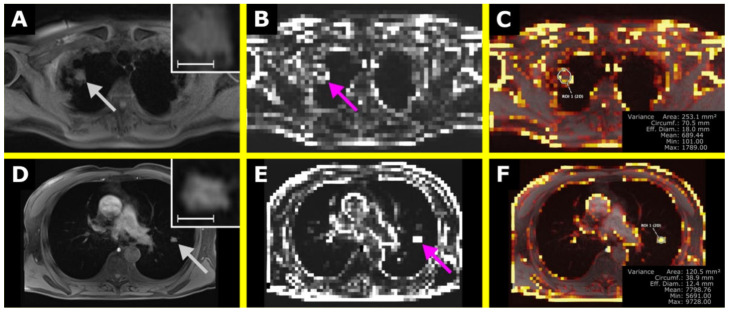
The upper row shows a pulmonary lymphoma manifestation in the right upper lobe with the T1-weighted (T1w) image in (A) and the corresponding map of Variance in (B). In (C), an overlay was created, also showing a ROI to retrieve Variance within the lesion. The lower row shows a non-lymphoma infiltrate in suspected fungal pneumonia in the left upper lobe with the T1w image in (D), the corresponding map of Variance in (C), and the overlay in (F). In (A,D), the lesions are shown in a magnified section in the upper right corner. The scale indicates 1 cm. (E) is the corresponding map of Variance of (D). The VISAGE Viewer was used to create the overlay map and to perform the measurements (Visage Imaging Client 7.1.15; Visage Imaging GmbH, Berlin, Germany).
